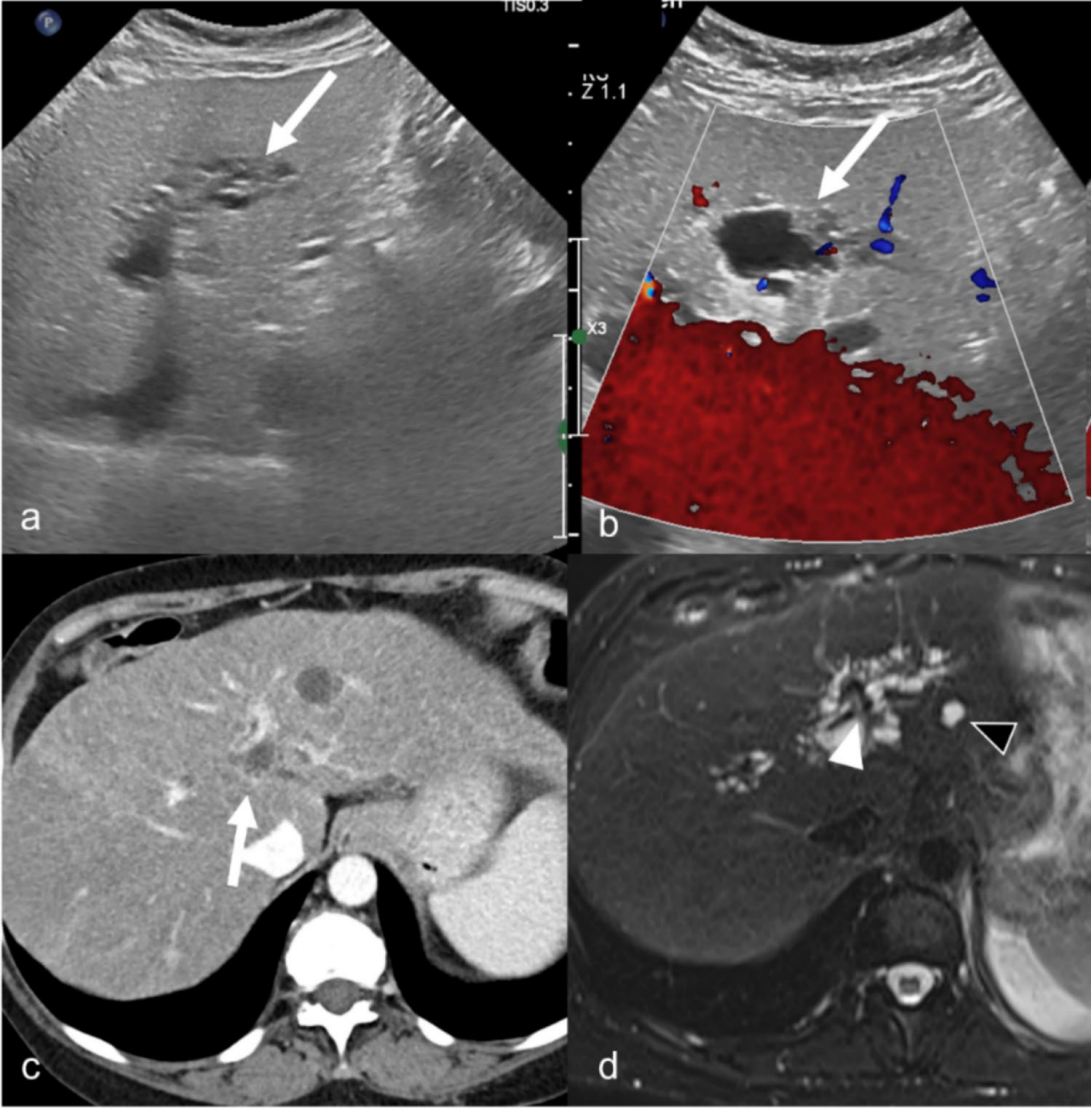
Peribiliary cyst in a 54-year-old female patient with a history of alcoholic cirrhosis and worsening of disease over time. Grayscale (a) and color Doppler (b) ultrasound images show cystic changes without flow on color Doppler (arrows). Axial contrast-enhanced CT shows cystic changes along the portal vein (arrow). MRI Axial T2-weighted fat-saturated (d) image shows multiple small T2-weighted hyperintense cysts along the portal vein (white arrowhead) and a simple cyst (black arrowhead). The MRI better depicts the extent of peribiliary cyst than the other imaging modalities
